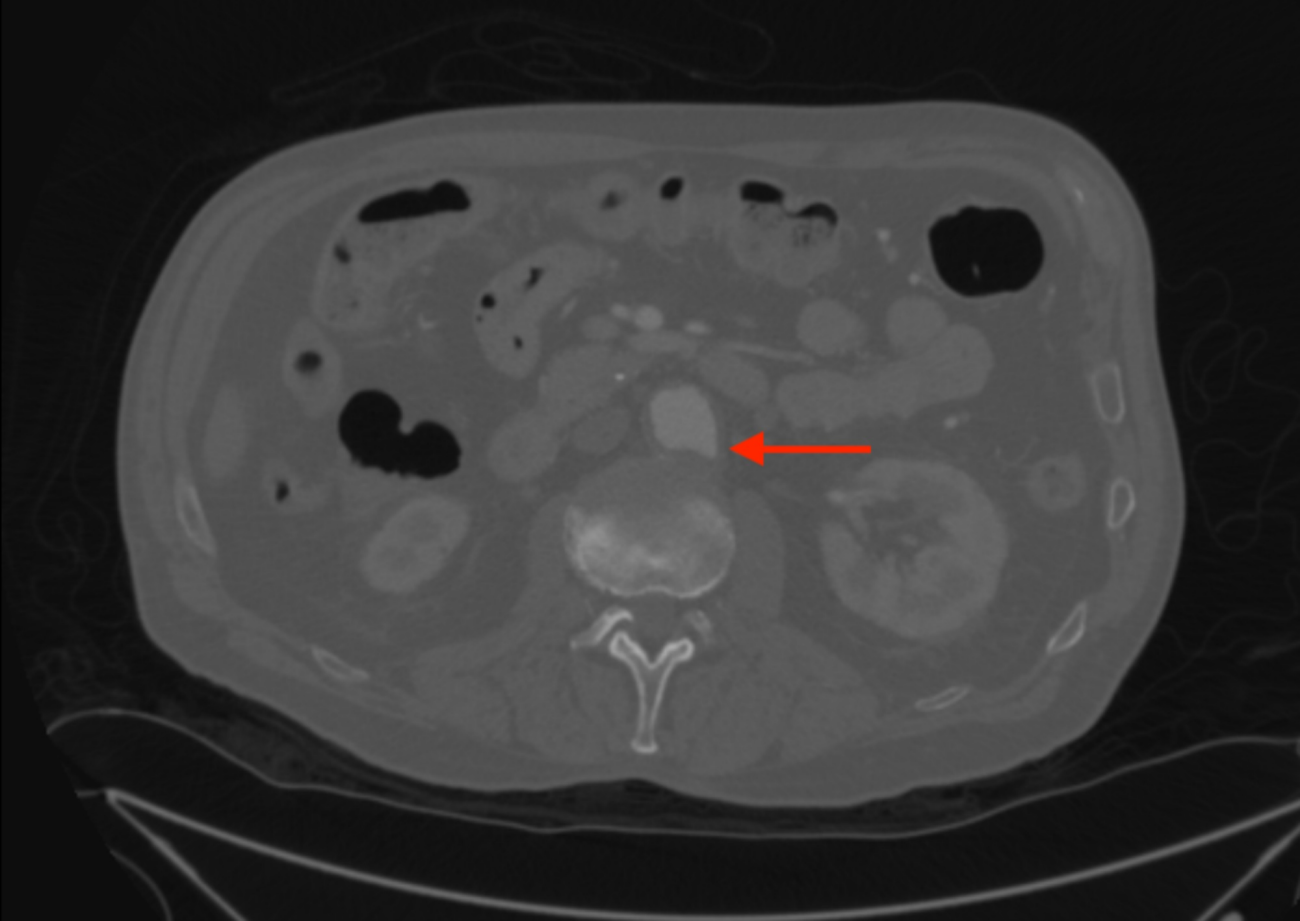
CT reveals a 5-mm penetrating aortic ulcer and 3.6-mm aneurysm of the infrarenal abdominal aorta (arrow).
